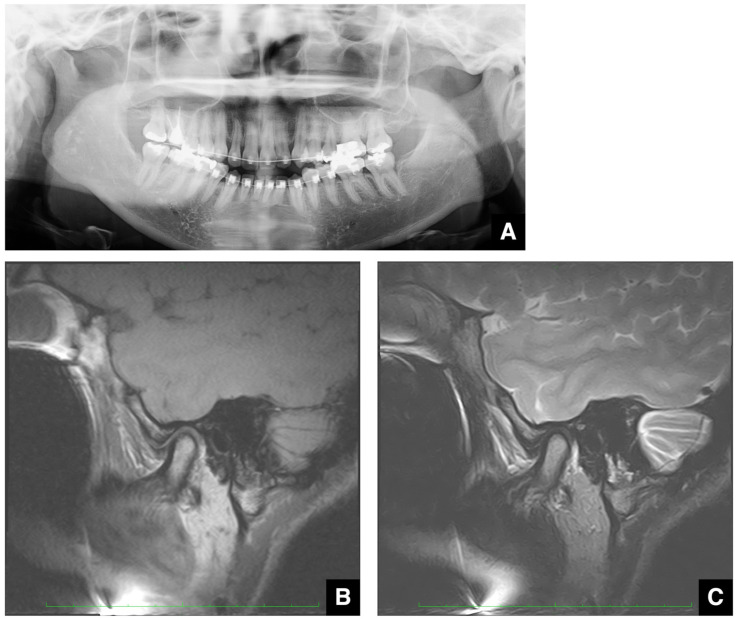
Images of a patient undergoing orthodontic treatment with archwires in place. (A) Panoramic radiograph shows the archwires. (B) T1-weighted MR image (TR 1050 ms; TE 18 ms; slice thickness 3.0 mm) of the same patient as in (A). (C) T2-weighted MR image (TR 4000 ms; TE 108 ms; slice thickness 3.0 mm) of the same patient as in (A). In both the T1- and T2-weighted images, artifacts obliterate the jawbone region but do not affect the visualization of the temporomandibular joint. Permission has been obtained to publish all figures.
